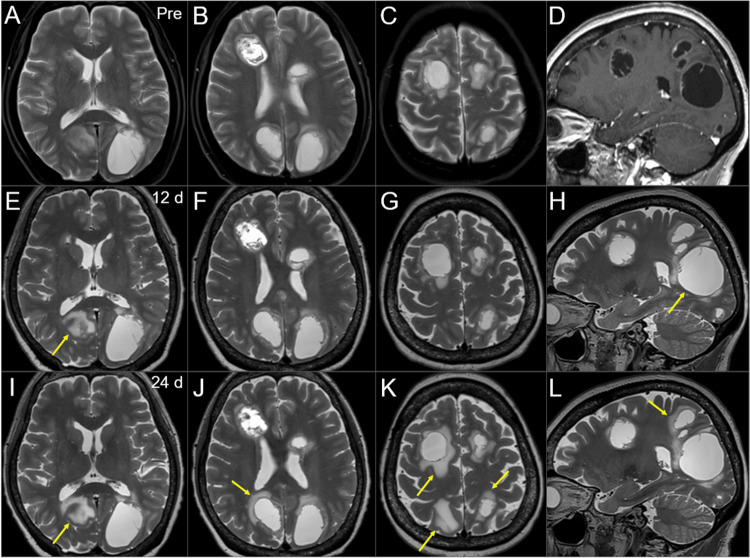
Magnetic resonance images during the 15-fr radiosurgery and subsequent WBRT. The images show T2-WIs (A-C, E-L); a CE T1-WI (sagittal T2-WIs before WBRT were unavailable) (D); axial images (A-C, E-G, I-K); sagittal images (D, H, L); three days before the initiation of WBRT (pre) (A-D); 12 days (d) after the WBRT initiation (at the 8 fr of WBRT) (E-H); and 24 days after the WBRT initiation (at the completion of SRS boost) (I-L). (A-H) These images are shown at the same magnification and coordinates under co-registration and fusion based on the pre-SRS images. (E-H) There was no obvious change in the tumor configuration of most lesions. The largest lesion was slightly enlarged (arrow in H). The perilesional edema aggravated in some lesions (arrow in E). (I-L) There is still no obvious change in the tumor configuration of almost all the lesions. The expansion of the largest lesion has not progressed. The perilesional edemas appear or aggravate in multiple lesions (arrows in I-L). WIs: weighted images; CE: contrast-enhanced; WBRT: whole-brain radiotherapy; SRS: stereotactic radiosurgery; fr: fraction
